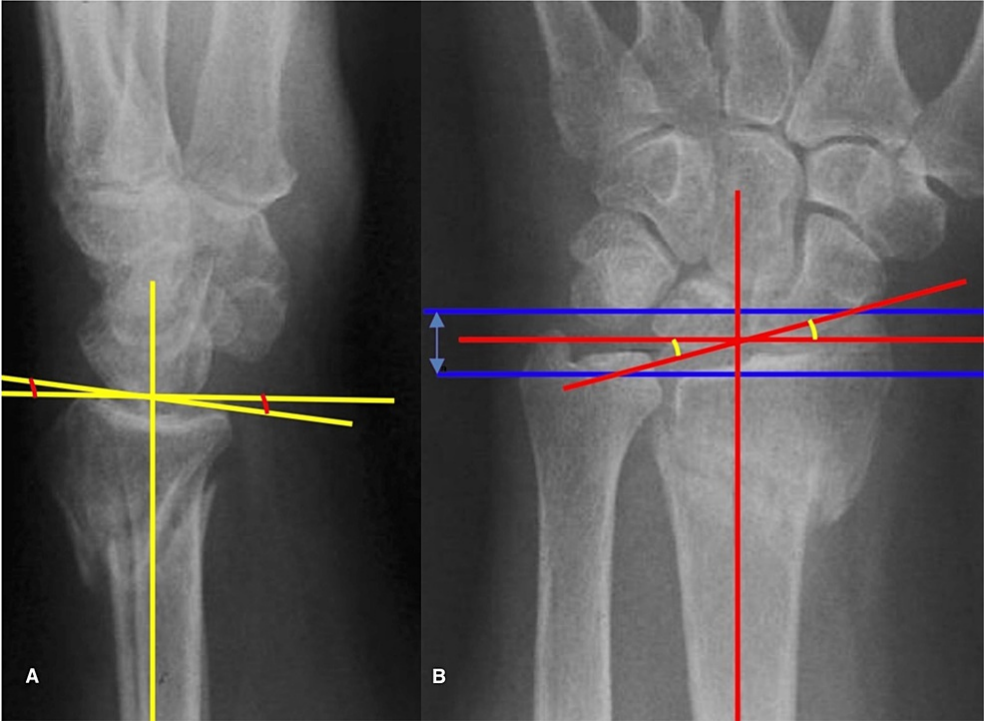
Radiographic measurements of the distal radius. (A) Lateral view of the wrist joint and the yellow lines for the measurements of Radial tilt. (B) Anteroposterior view of the wrist joint. Blue lines are for measurement of the radial length, red lines for the measurement of radial inclination.
